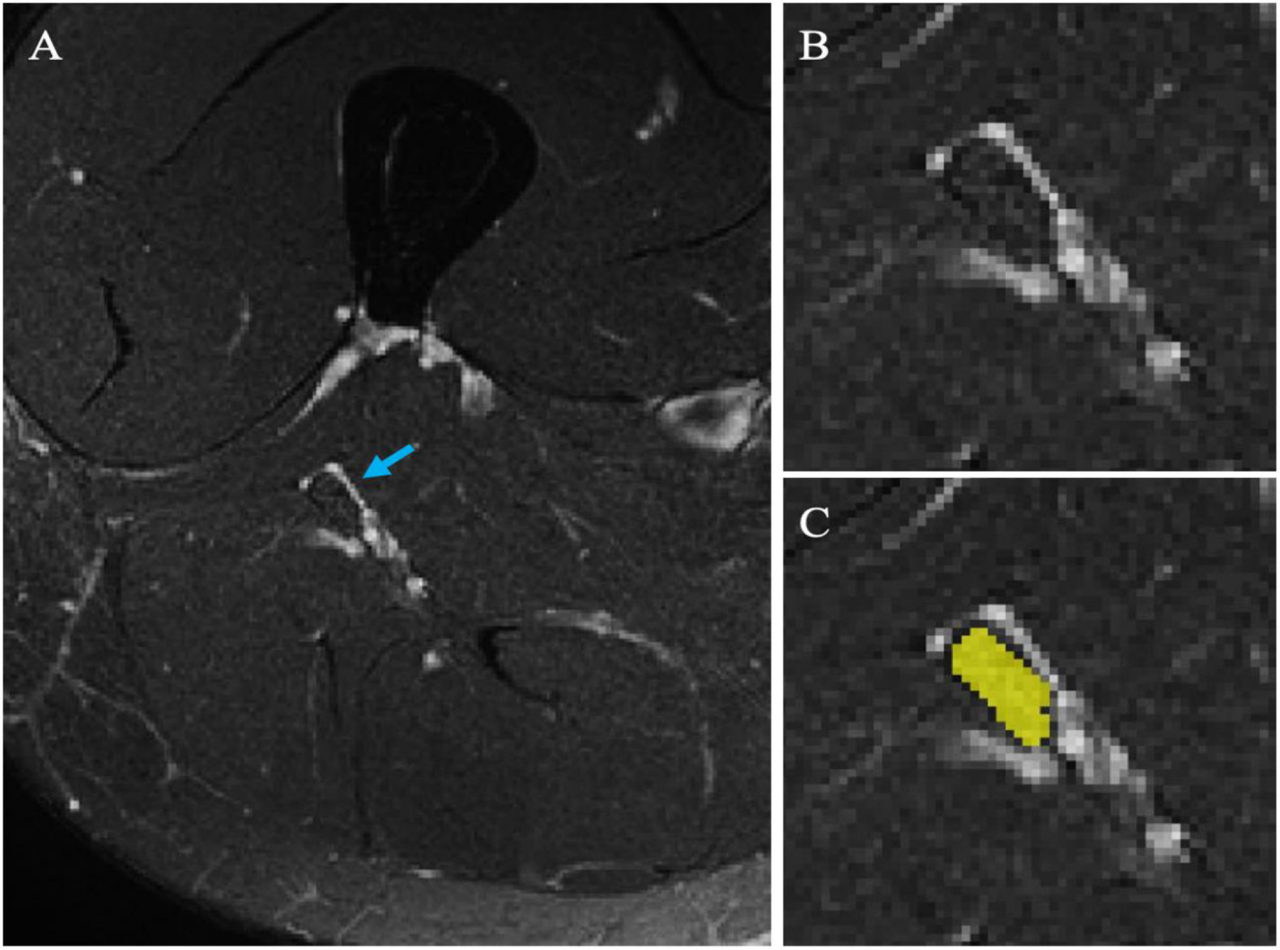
(A) Example image of the sciatic nerve (blue arrow) obtained using the high-resolution fat-suppressed T2-weighted sequence; (B) Magnified image of the sciatic nerve shown on the left; (C) Manual segmentation of the sciatic nerve (binary mask shown in yellow).
